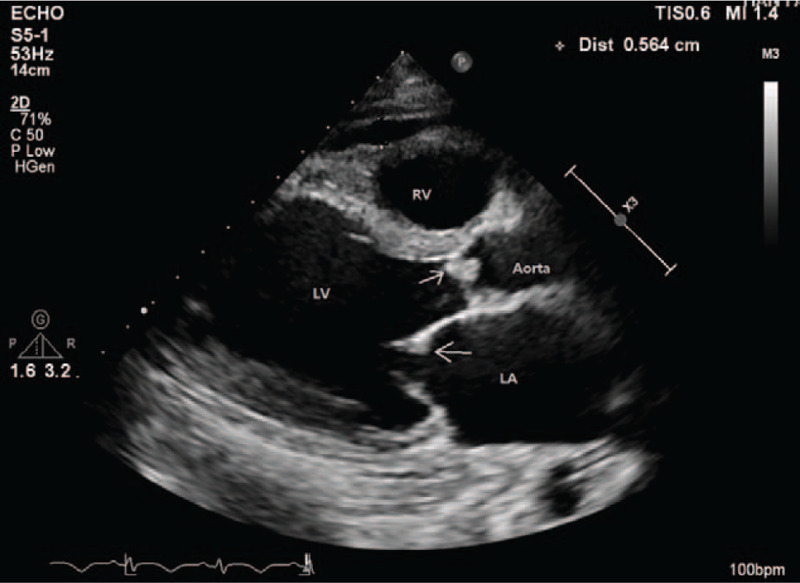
Echocardiographic findings before surgery. In the parasternal long-axis view, the arrow indicates significant vegetations in aortic and mitral valves. LA = left atrium, LV = left ventricle, RV = right ventricle.
